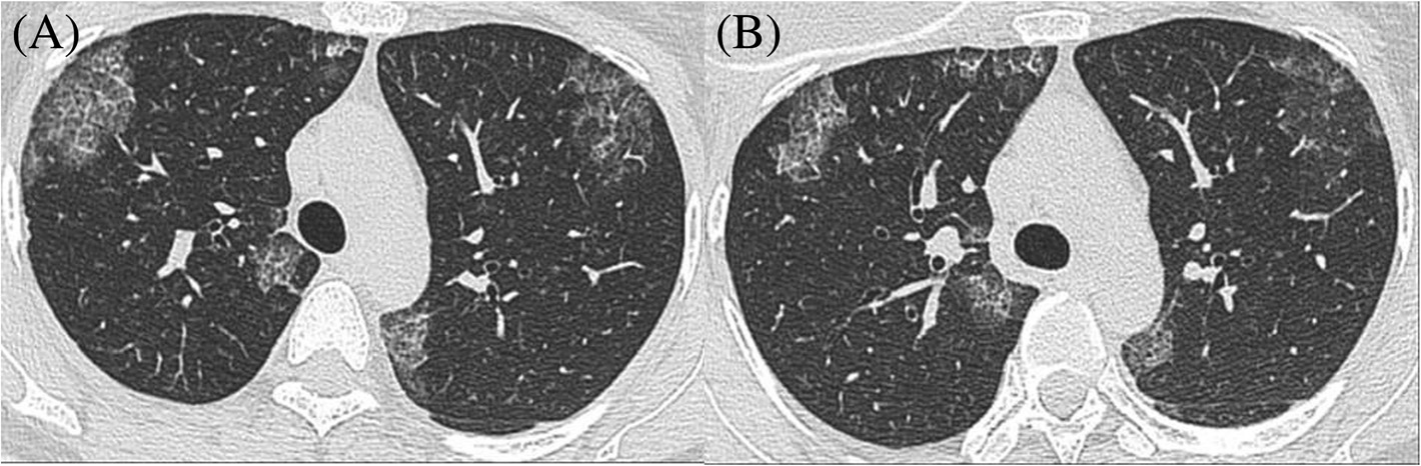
Serial chest computed tomography shows gradual resolution. (A) The chest computed tomography scan shows patchy peripheral ground-glass opacities and thickened interlobular septa in both upper lung fields (November 2013). (B) The chest computed tomography scan shows that the areas of ground-glass opacities and thickened interlobular septa representing pulmonary alveolar proteinosis have decreased (January 2014).
